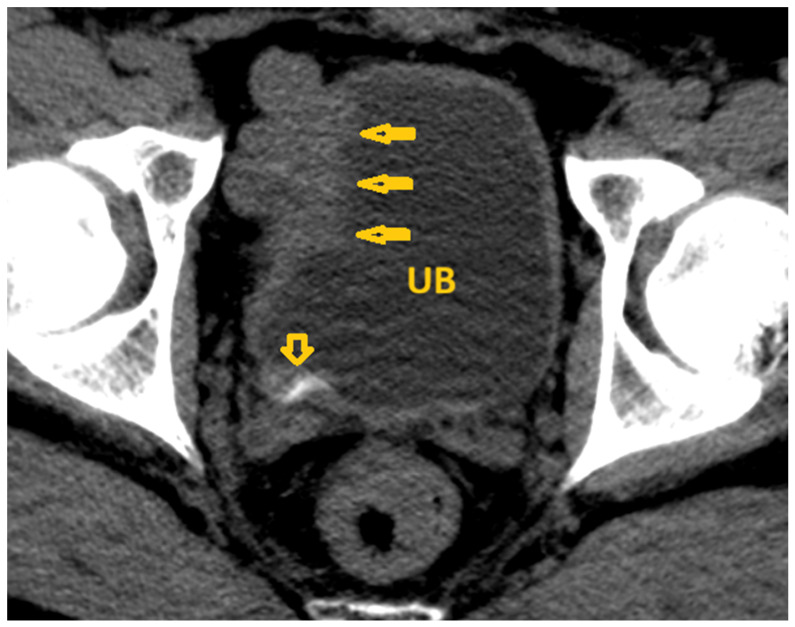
Selected non-contrast computed tomography axial image of a 27-year-old male patient shows polypoidal thickening of the right urinary bladder wall (long arrows) with small calcification seen at the right distal ureter, reflecting *Schistosoma* cystitis (short arrow). UB: Urinary bladder.
